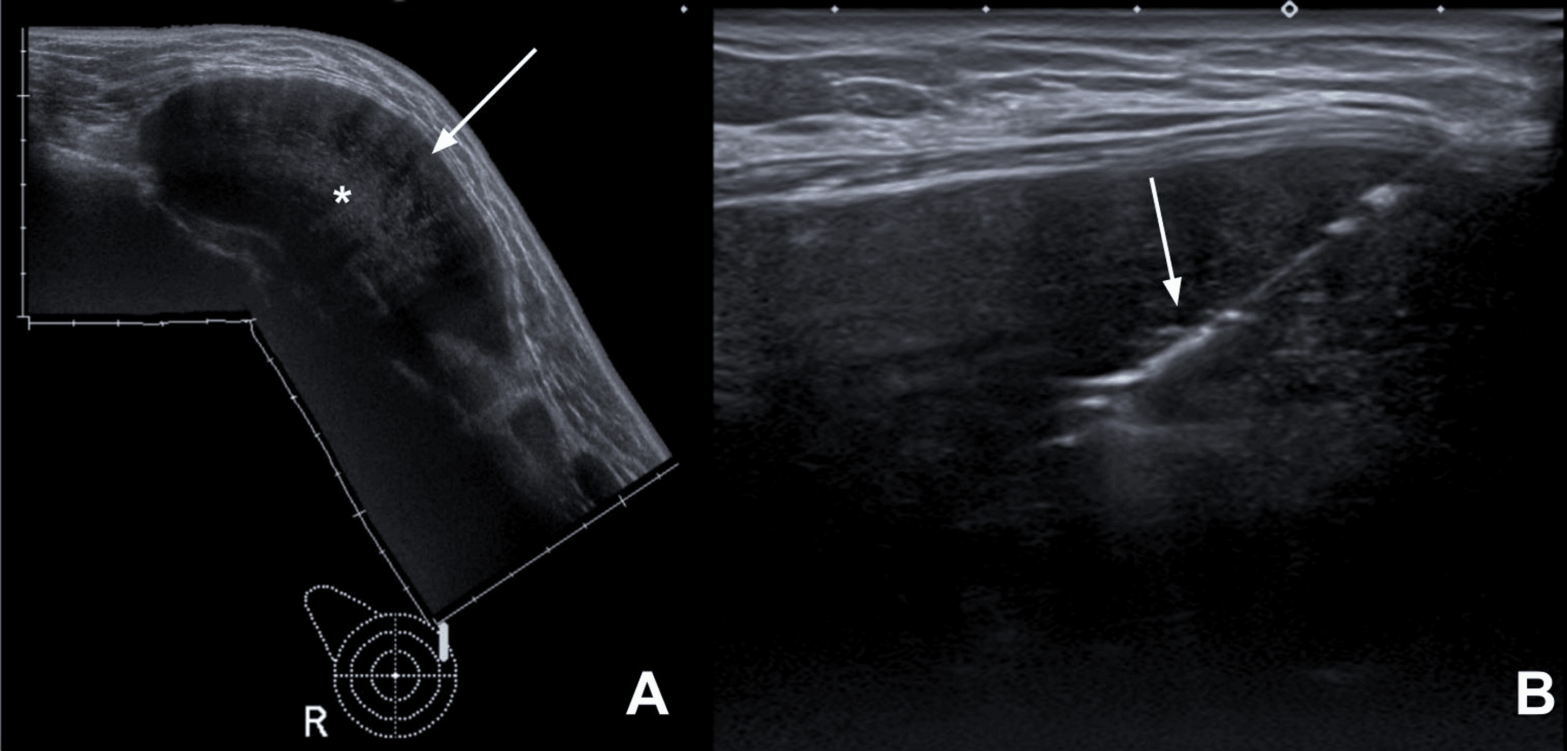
(A) Panoramic ultrasound image demonstrates a heterogeneous lesion located in the posterior region, medial to the breast prosthesis in the right rib cage. The lesion is predominantly hypoechoic (arrow) with a centrally located echogenic component (*). (B) A guided biopsy of the lesion was performed (trucut needle shown with the arrow).
